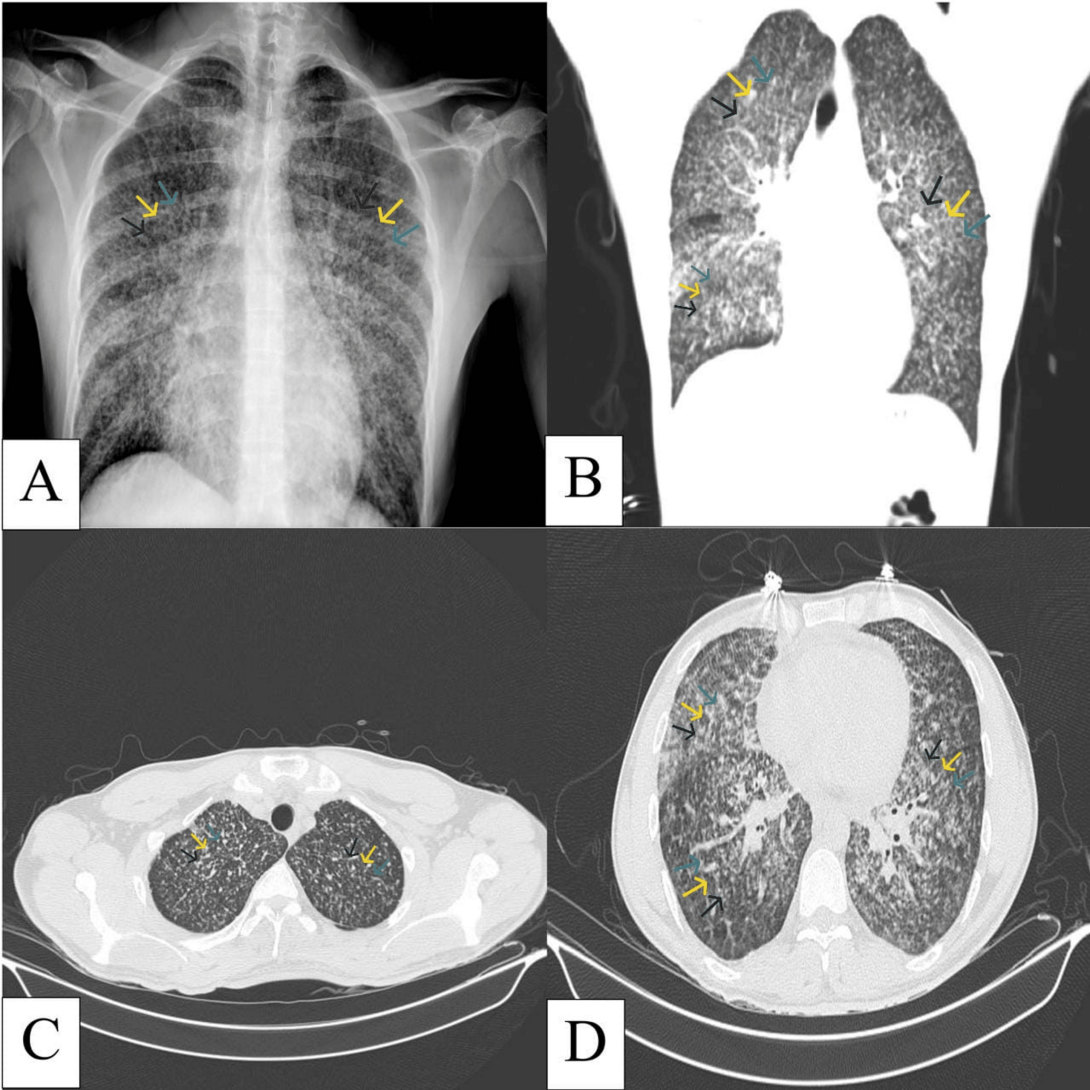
Chest radiography and CT images (A) Chest X-ray showing increased bronchovascular markings (arrows). (B) Coronal CT image of the chest showing diffuse pulmonary nodules measuring 1-2 mm (arrows). (C) Axial CT image showing diffuse pulmonary nodules in both upper lobes (arrows). (D) Axial CT image showing diffuse pulmonary nodules in the right middle and lower lobes, lingula, and left lower lobe (arrows).
